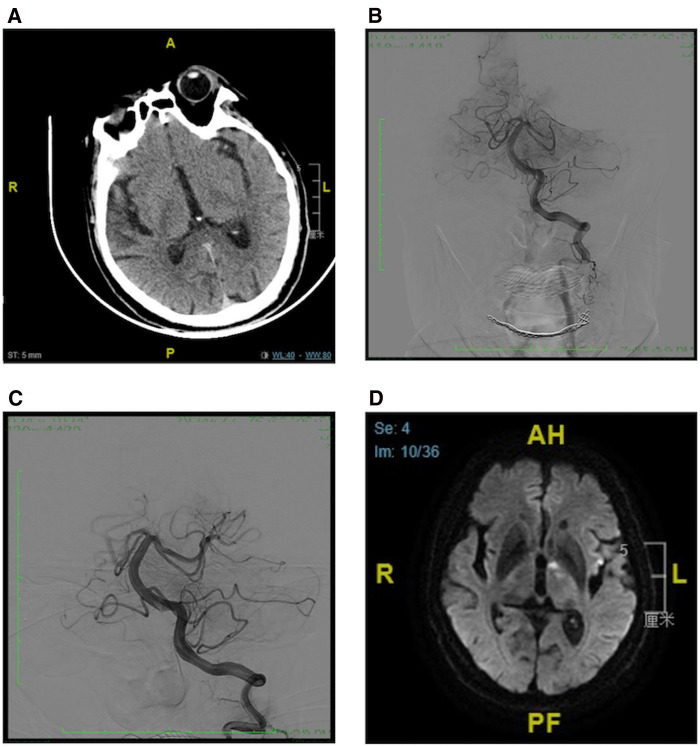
Case 2. (A) Baseline CT indicated pc-ASPECTS score was 10, (B) Anterior-posterior angiogram revealed an occlusion of left PPCA. (C) Post-thrombectomy angiogram indicated the mTICI score was 2b. (D) Post-procedural DWI showed acute left thalamic infarction. PPCA, proximal posterior cerebral artery; pc-ASPECTS, posterior circulation Alberta Stroke Program Early CT Score; mRS, modified Rankin Scale.
